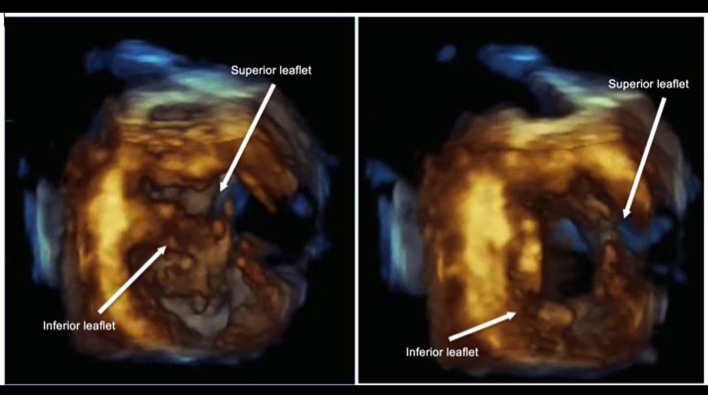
3D echocardiography demonstrating a common AV valve during systole (left) and diastole (right) taken at short axis view with its corresponding M-mode. No significant regurgitation was noted on color flow Doppler.
